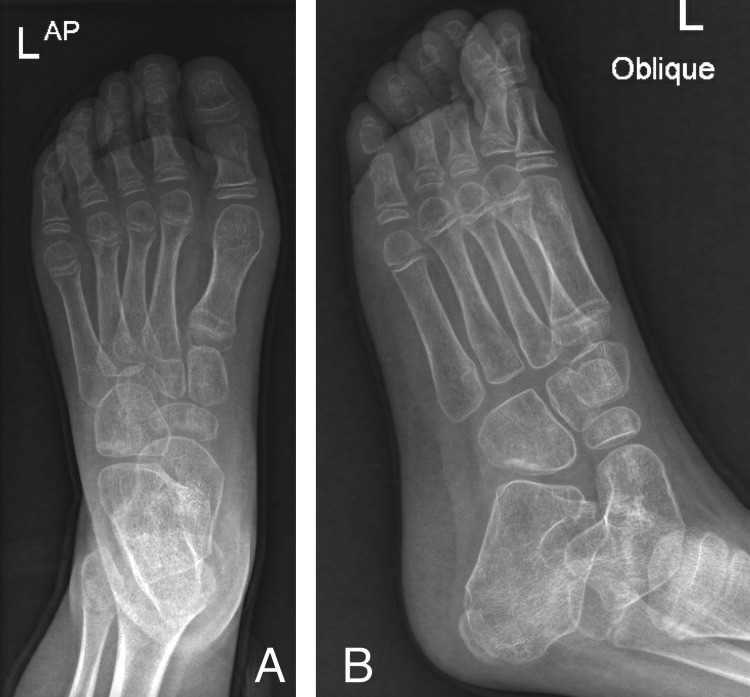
Postoperative X-ray of the left side Postoperative anterior-posterior (A) and oblique (B) X-ray of left ankle showing normal ankle alignment.
